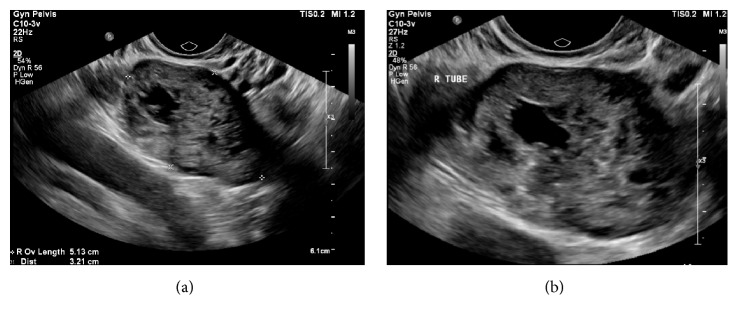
Ultrasound images showing normal right ovary and right tubal ectopic.
